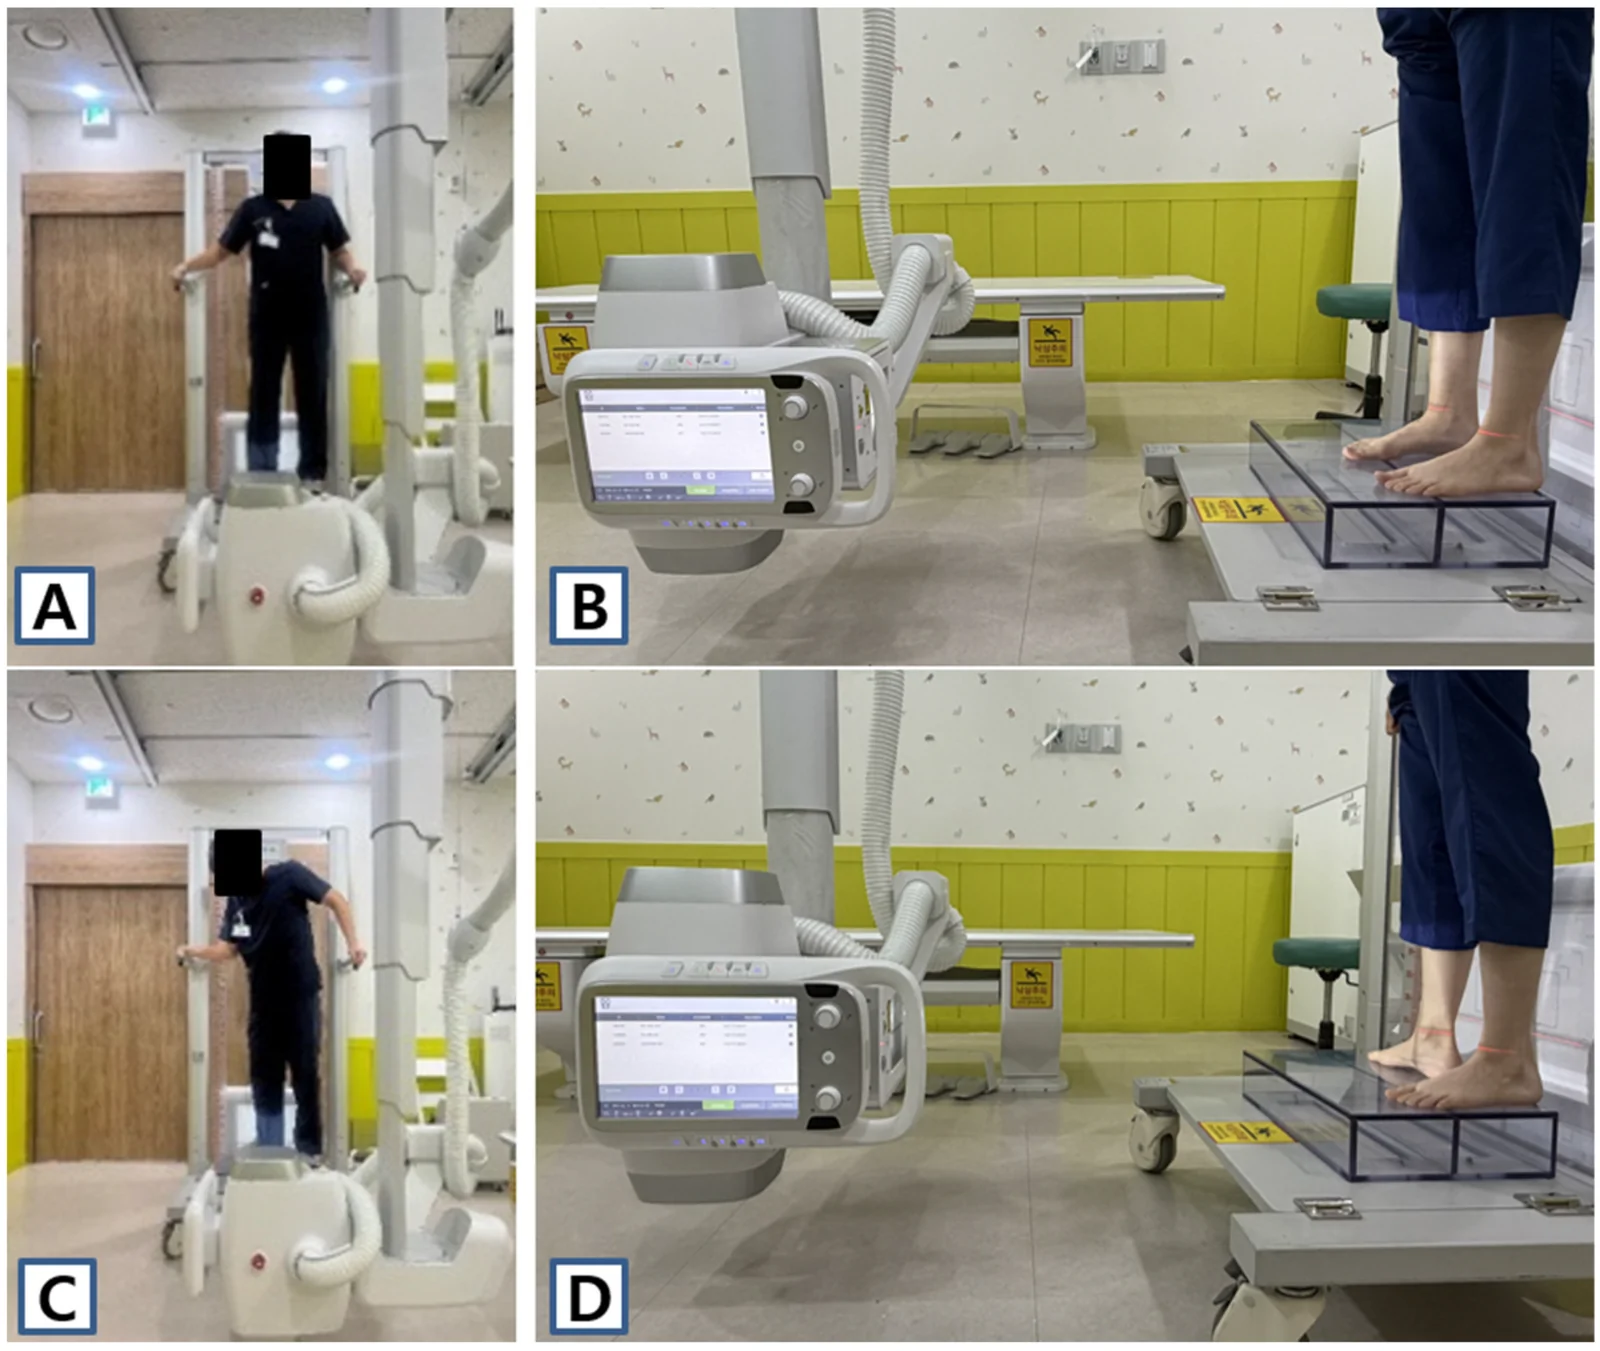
Standing ankle AP/Lat radiographic acquisition techniques. (A,B) demonstrate the frontal and lateral positions of the X-ray beam projector, respectively, during standing ankle AP radiograph. (C,D) show the frontal and lateral positions of the X-ray beam projector, respectively, during standing ankle lateral radiograph.
